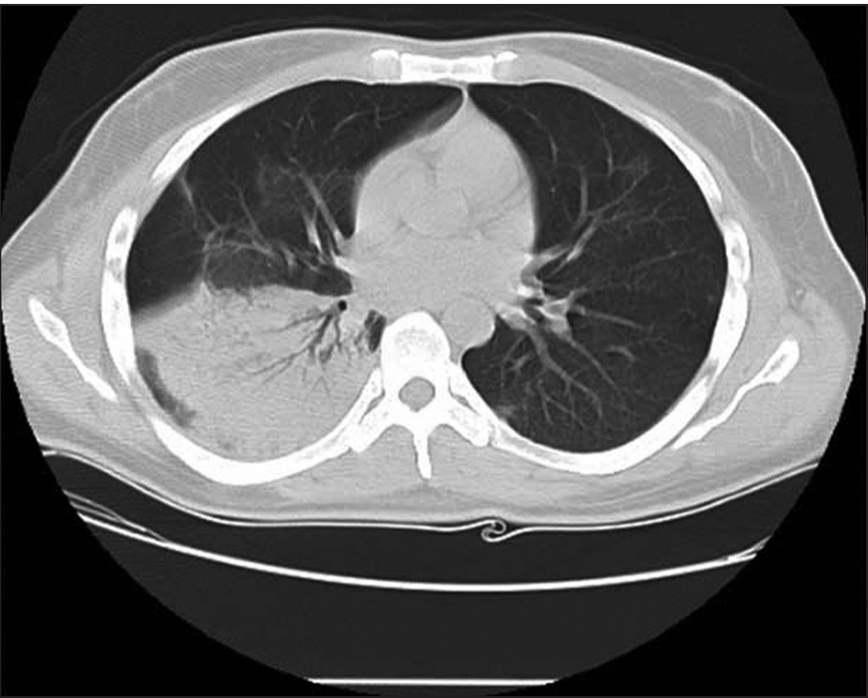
Chest CT scan, three weeks after presentation, showing persistent right lower lobe consolidation.
